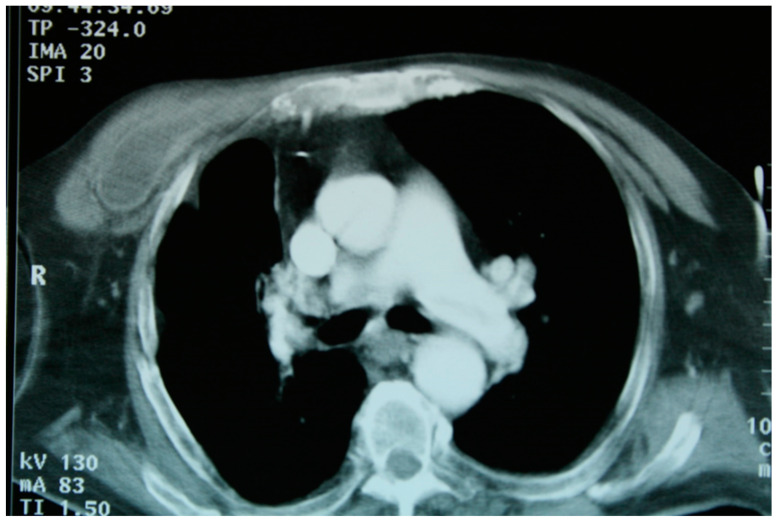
A chest CT of patient 2. A lesion misdiagnosed as a soft-tissue abscess that was excised in a similar manner as the previous case.
